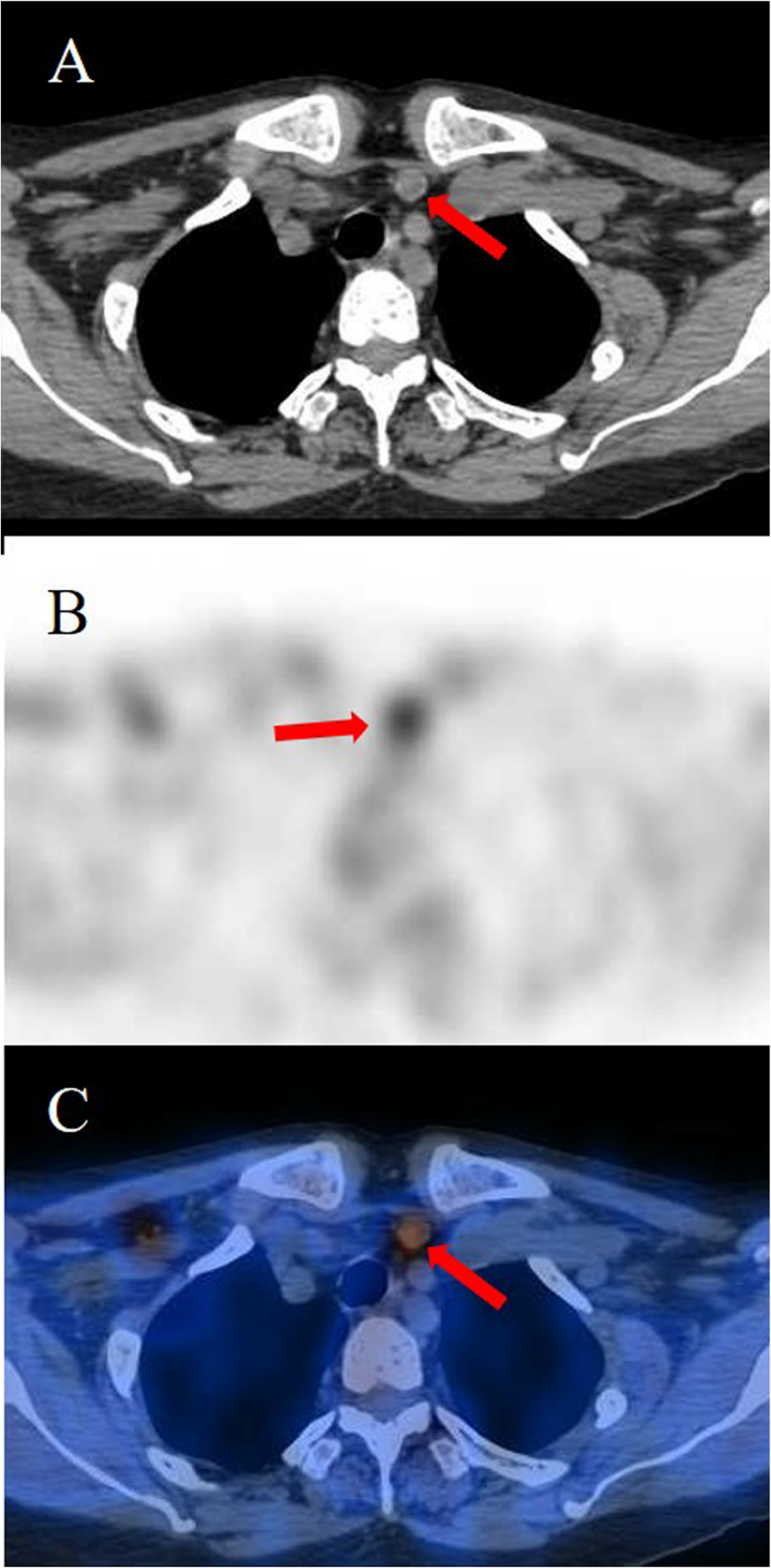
The ^99m^Tc-MIBI SPECT/CT image indicates a low-density lesion with high tracer uptake at the site of the inferior clavicle head (red arrows: A, axial image; B, SPECT; C, fusion image)
